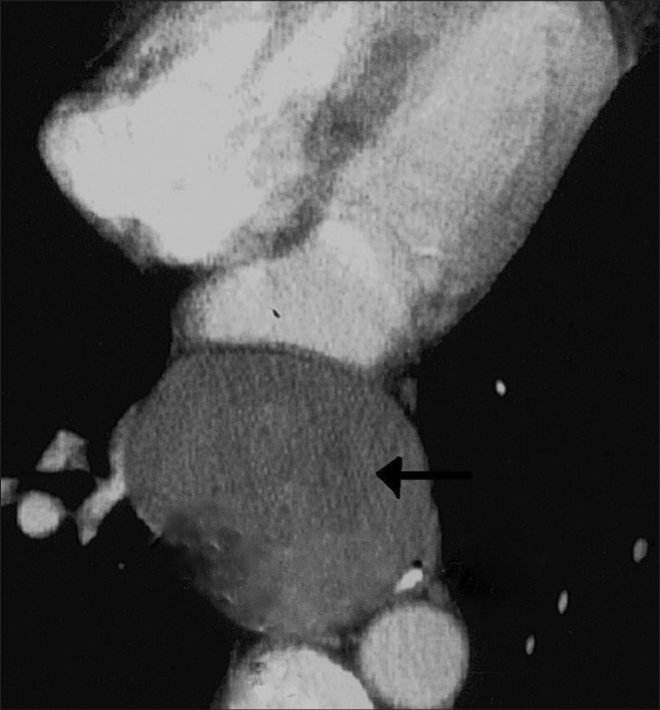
Contract enhanced chest CT scan shows 9.5 × 6.5 × 6 cm well defined homogenous cystic lesion (arrow) having attenuation values of 0-20 HU in the middle mediastinum encroaching upon the posterior mediastinum and compressing the esophagus
